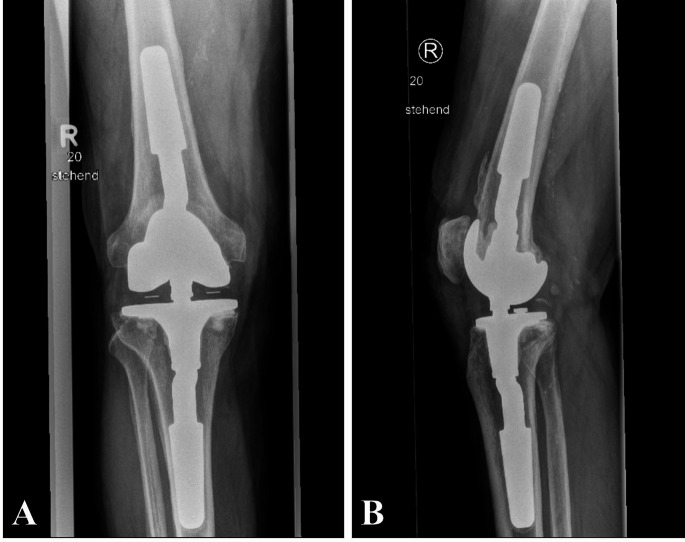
Preoperative X-ray images in two planes before DAIR Procedure (A: a.p.; B: lateral)
